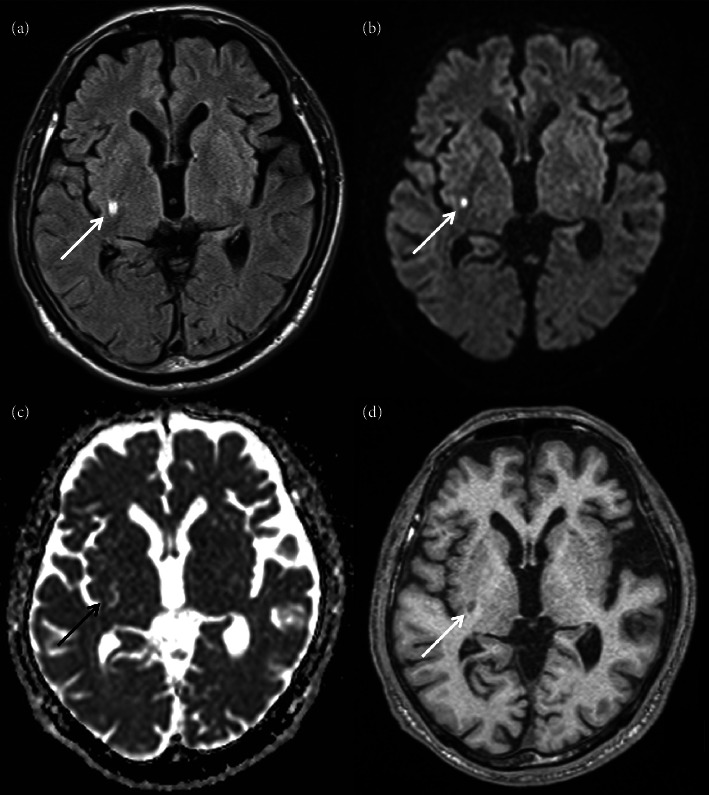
Head magnetic resonance imaging findings. Fluid-attenuated inversion recovery imaging revealed a hyperintensity lesion in the right lenticular nucleus and internal capsule (arrow, (a)). The lesion was partly hyperintense on diffusion-weighted imaging (arrow, (b)) and corresponded to an isointense area accompanied by an adjacent irregular hyperintensity on apparent diffusion coefficient map (arrow, (c)). Moreover, on T1 images, it was largely hypointense (arrow, (d)) in the absence of striatal hyperintensities.
